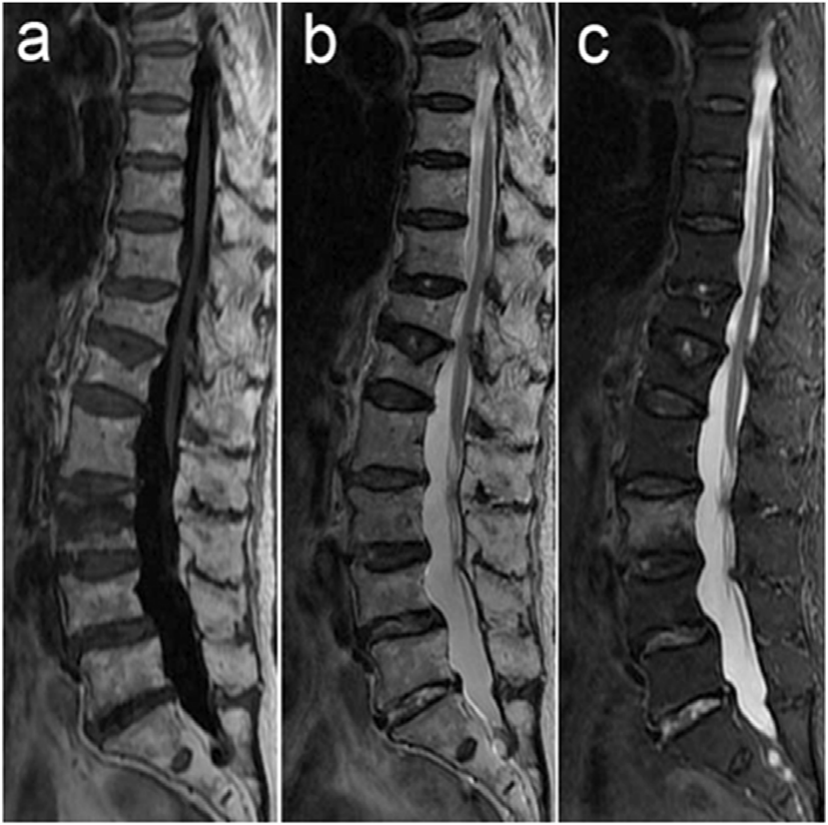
Preoperative MRI examination. L3 vertebral body showed low signal (a), T2 and other signals (b) and STIR images showed a high signal (c). This confirmed L3 as the vertebral body responsible for the pain.
